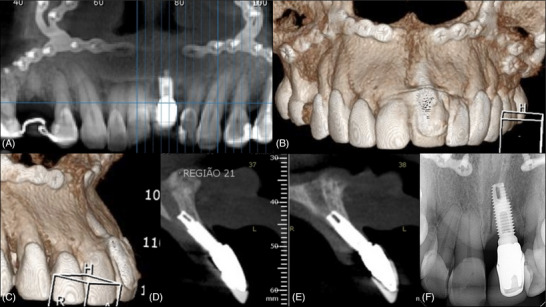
Case 3: Initial radiographic evaluation. (A) Panoramic reconstruction showing the implant in the region of tooth #9. (B) 3D reconstruction reveals the implant's buccopalatal volume and the significant bone deficiency in the region. The presence of fixation plates from a previous orthognathic surgery is also noted. (C) A lateral view of the 3D reconstruction confirms the vestibular positioning of the implant and the associated bone deficiencies. (D–E) Sagittal cross‐sectional views demonstrating the buccal angulation of the implant, associated with both buccal and palatal bone loss, and its proximity to the nasopalatine canal. Additionally, the apical bone morphology appears unfavorable, with limited dimensions. (F) Periapical radiograph showing that, despite the absence of buccal and palatal bone plates, the interproximal bone levels remain satisfactory.
